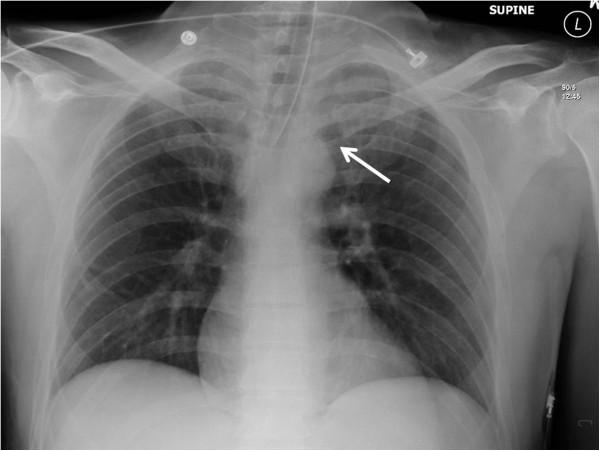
On-table chest radiograph after the revision procedure was obtained to rule out an iatrogenic left-side pneumothorax. The arrow denotes the resection arthroplasty of the left sternoclavicular joint.
